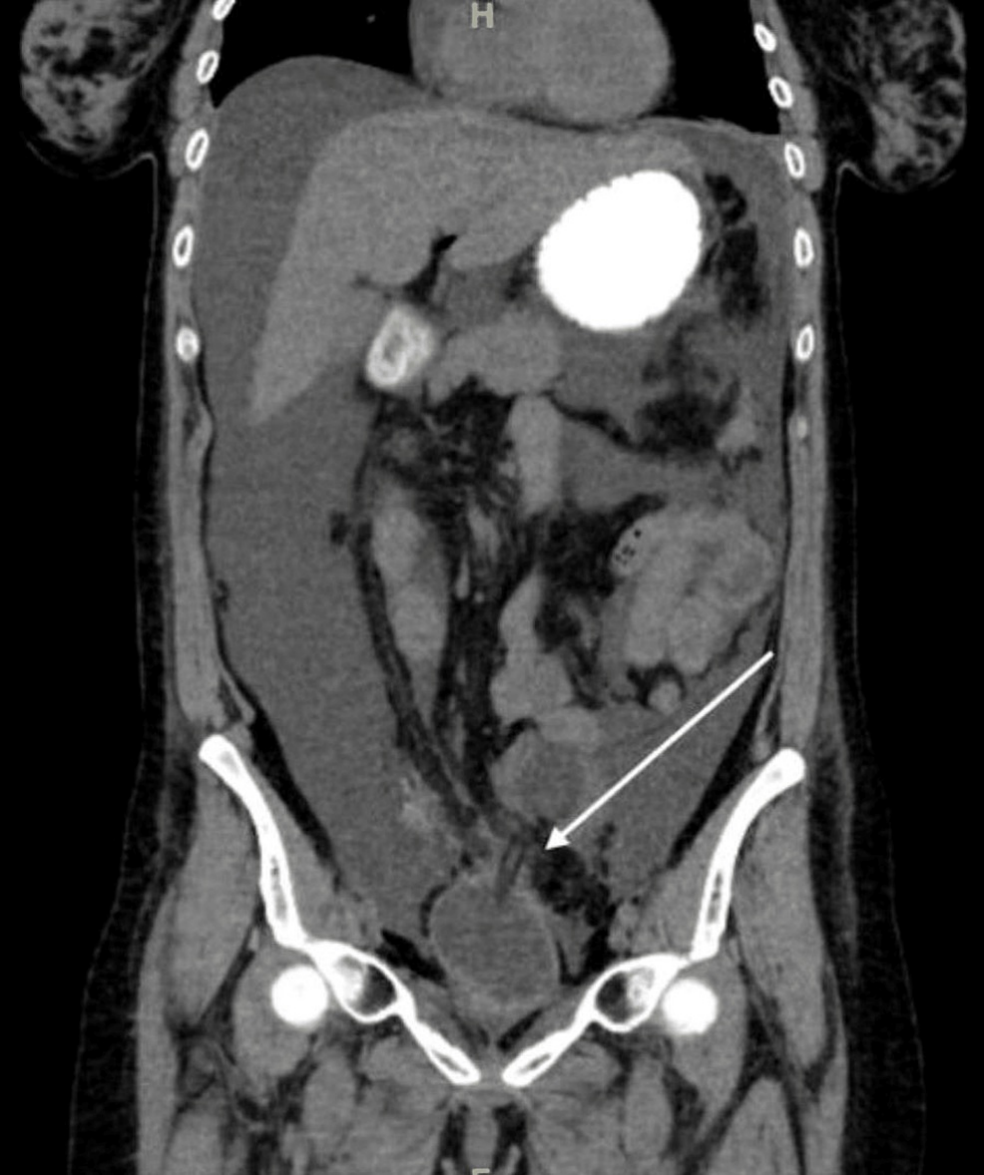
CT abdomen and pelvis without IV or oral contrast The coronal view demonstrates the Foley catheter tip (white arrow tip) extending beyond the urinary bladder dome wall with anterior pneumoperitoneum and perihepatic/colonic ascites.
